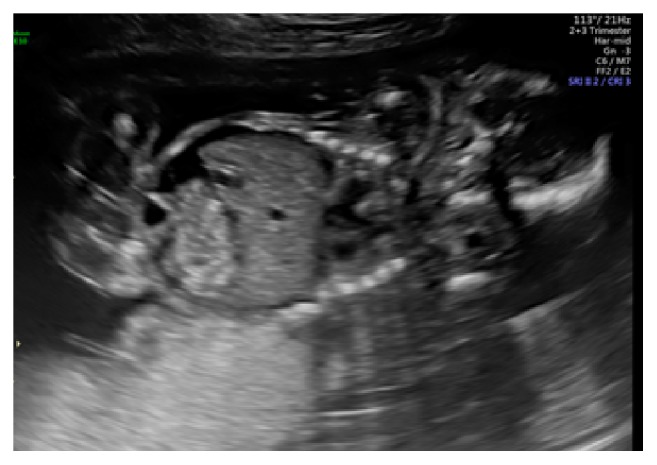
Anatomy ultrasound at 20 weeks' and 1-day gestation with fetal ascites.
